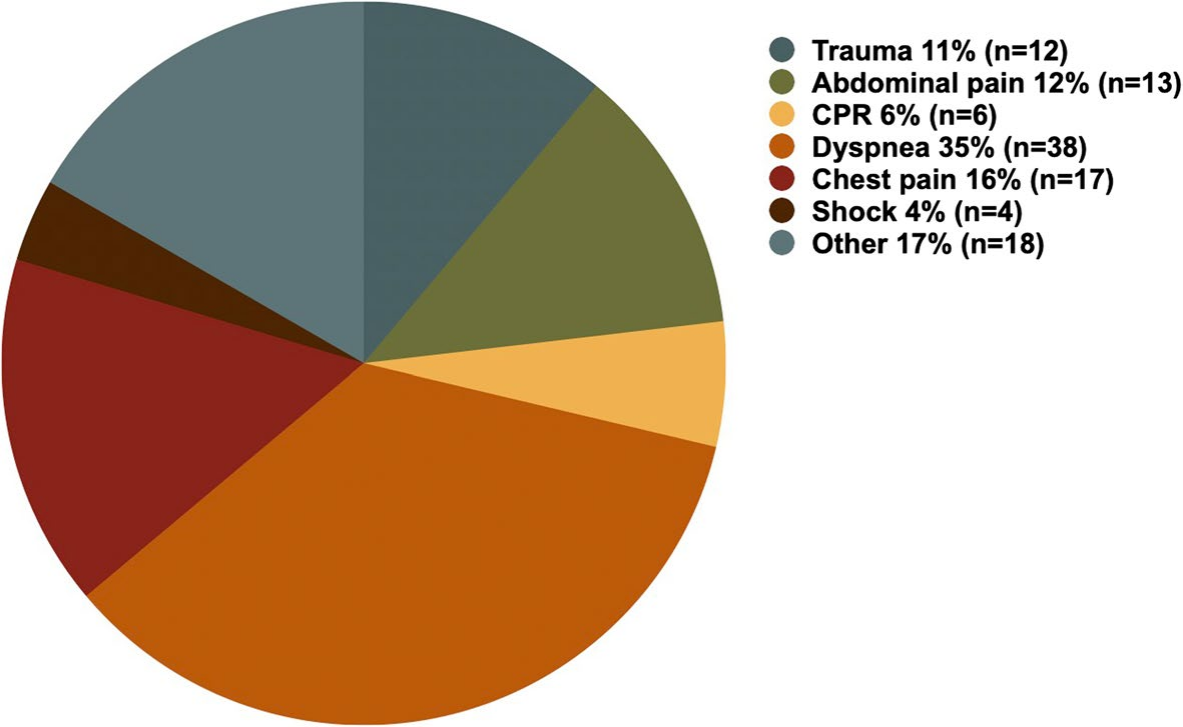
Indications for use of prehospital ultrasound diagnostics (other n=18: iv access n=6, not named n=12)
